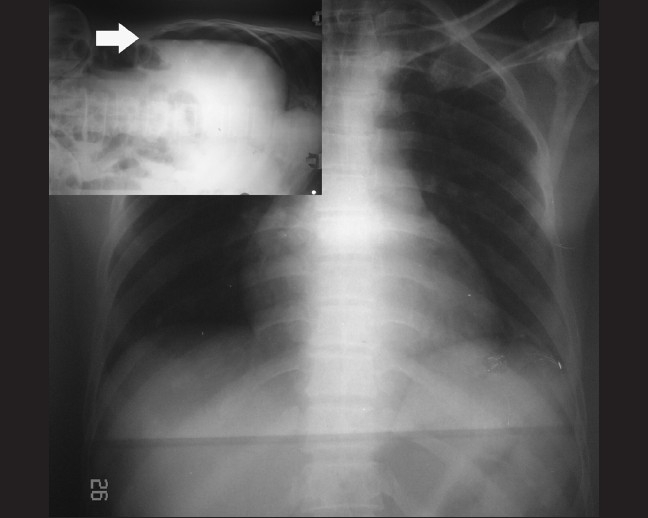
X-ray of the chest and upper abdomen with both the domes of the diaphragm appearing apparently normal. However, X-ray of the left lateral decubitus showed free gas in the peritoneum (inset, arrow)
